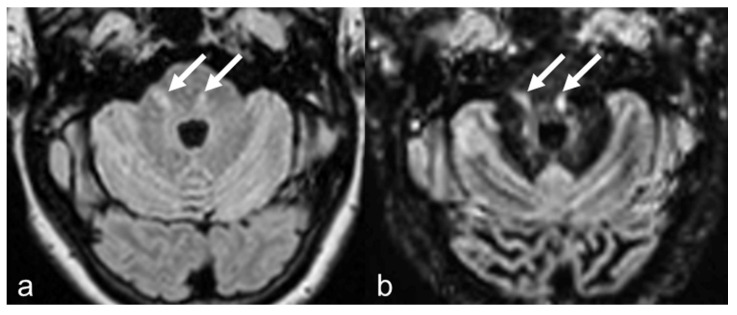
The pontine lesions (arrows) are well detected both on FLAIR (a) and on DIR images (b).
